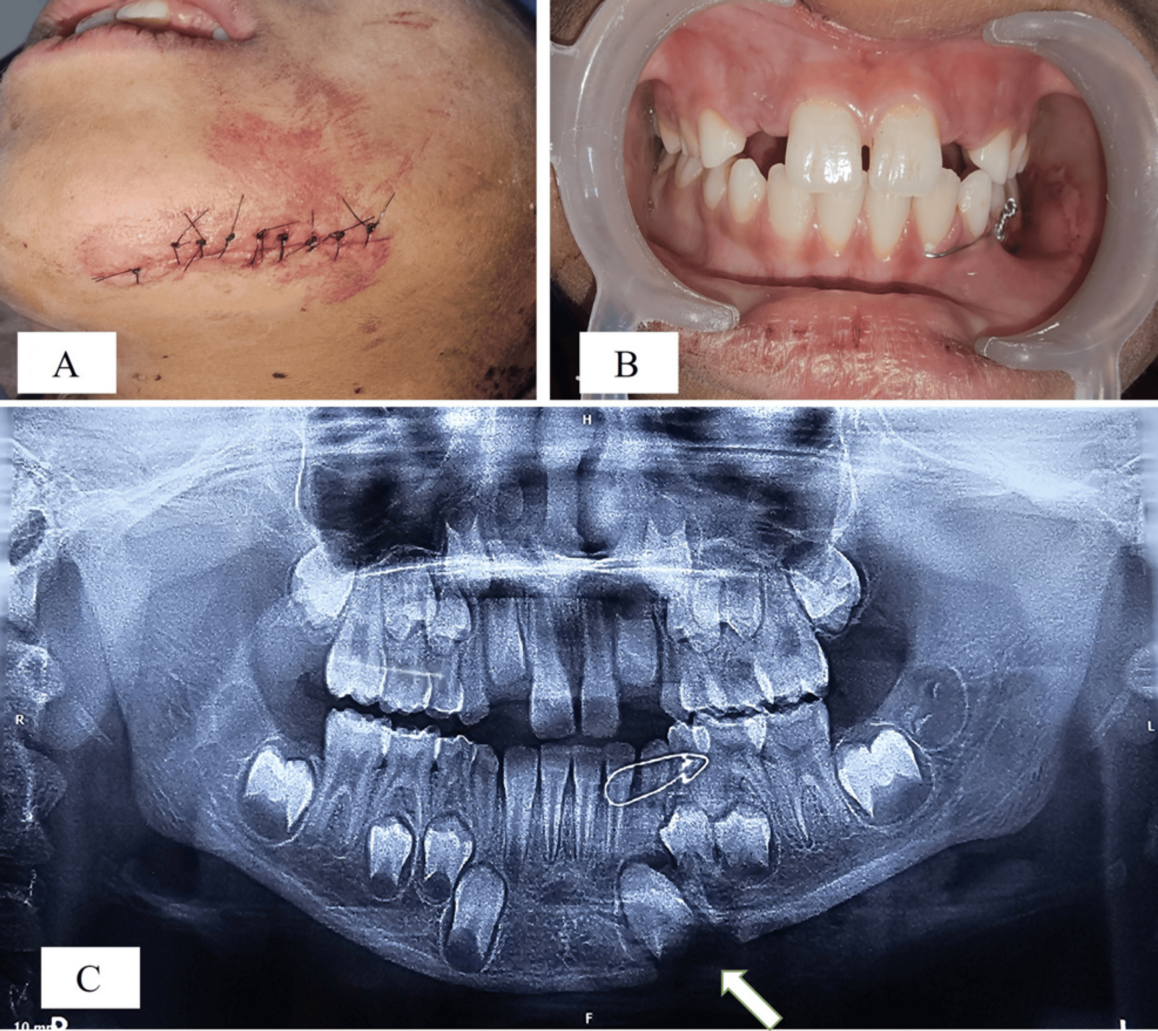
(A) Double-layered suturing with Vicryl 4-0 and Nylon 4-0; (B) intraoperative clinical photograph showing the proper occlusion after bridle wiring; (C) orthopantomogram (OPG) showing the bridle wiring and lost triangular bone fragment near tooth bud 33
